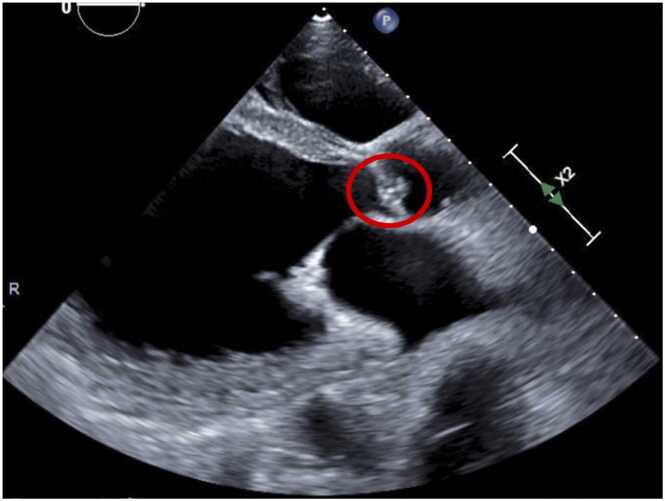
Transthoracic echocardiography showing a 5 × 4 mm vegetation on the aortic valve.
